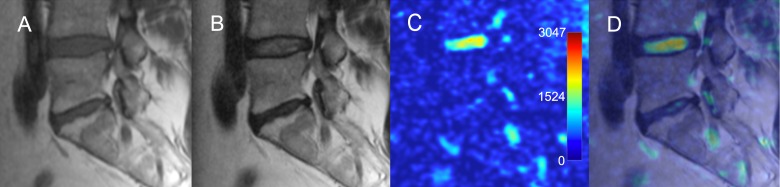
Modic type 2 changes of the vertebral bone marrow adjacent to a degenerated intervertebral disk. (A) T1-WI and (B) T2-WI show hyperintense signal. (C) ADC map and (D) overlay of ADC map over T2-WI show decreased signal intensity over the degenerated disk and over the area of reactive bone marrow changes. ADC, apparent diffusion coefficient; T2-WI, T2-weighted image.
